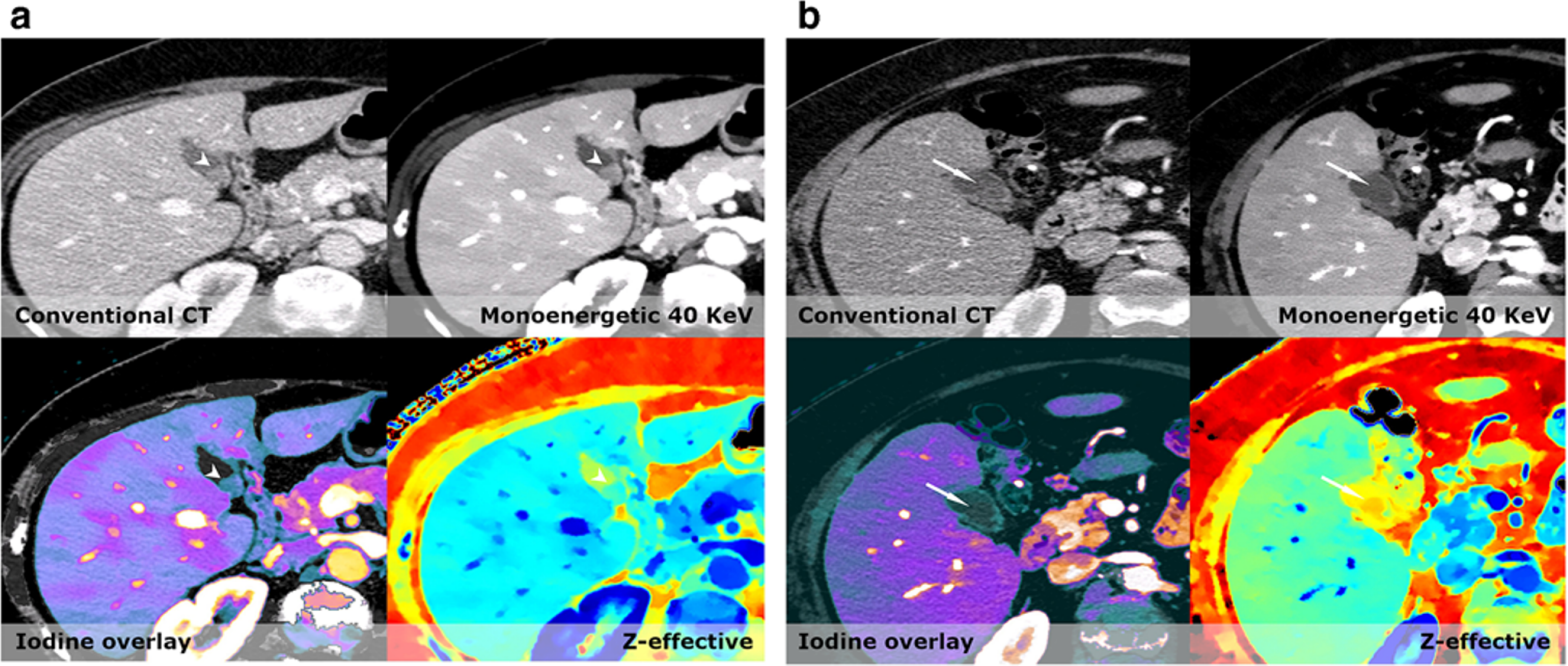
a Conventional CT shows a hyperdense process (arrowhead) in the gall bladder. The virtual low monoenergetic image increases the HU of the process; iodine density overlay shows increased levels of iodine around 1 mg/ml; *Z*-effective shows average atomic number close to the liver parenchyma. It could be an adenoma or adenocarcinoma; however, the patient did not wish to have further workup done. b Conventional CT show a slight hyperdense process in the gallbladder. Virtual low monoenergetic images make the lesion become hypodense, no iodine density is seen and *Z*-effective images show average atomic numbers closer to fat, giving us the diagnosis of a cholesterol stone
